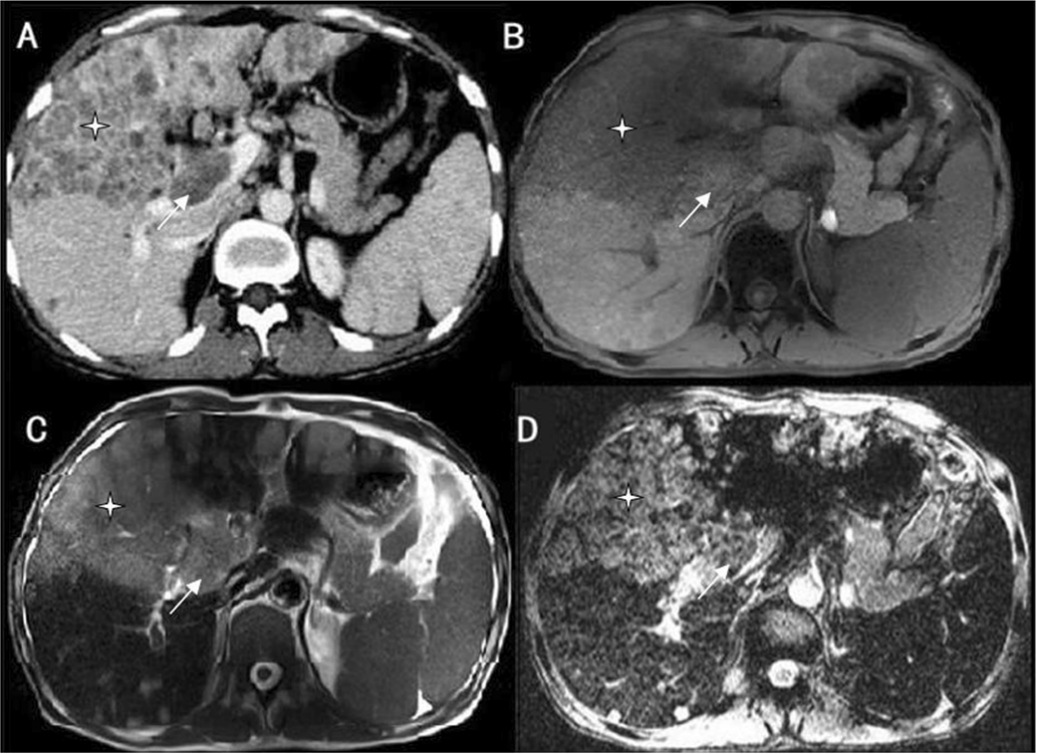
Neoplastic thrombosis of the portal vein in a patient with hepatocellular carcinoma (HCC). A: Contrast-enhanced multidetector computer tomography (CE-CT); B: T1-weighted imaging (T1WI); C: T2-weighted imaging (T2WI); and D: Susceptibility-weighted imaging (SWI). A large HCC (*) is seen in the right lobe of the liver and invades the right portal vein (white arrow). The HCC and portal vein thrombus display similar signal intensity (SI) by SW imaging.
